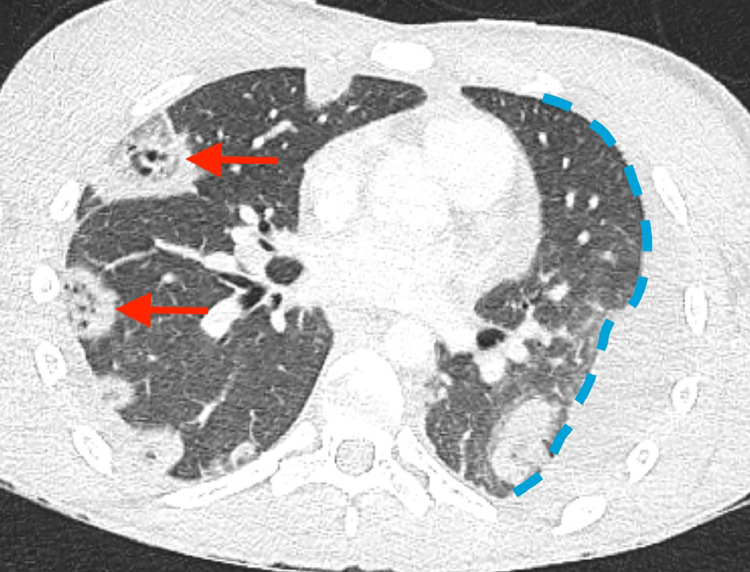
CT pulmonary angiogram lung window: multiple cavitating nodules, positioned both peripherally and centrally. Two of these are highlighted by red arrows. A loculated pleural effusion is also delineated in blue.
